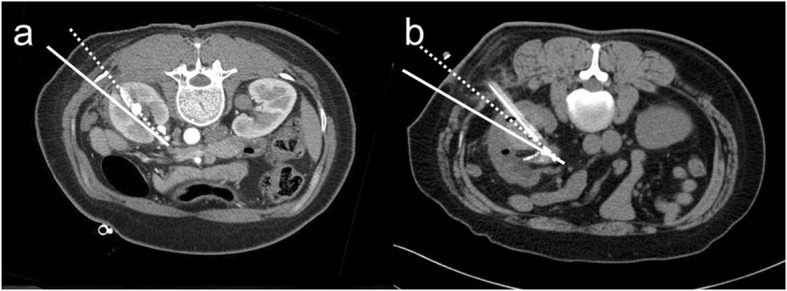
Example of a CT scan of a correct (a) and incorrect (b) puncture. Solid line represents the frontal plane, and dotted line represents the line 20 degrees posterior to the frontal plane. In the CT scan of correct puncture (a), the tract is punctured within Brödel’s line. In the CT scan of incorrect puncture (b), the tract is punctured outside of Brödel’s line
